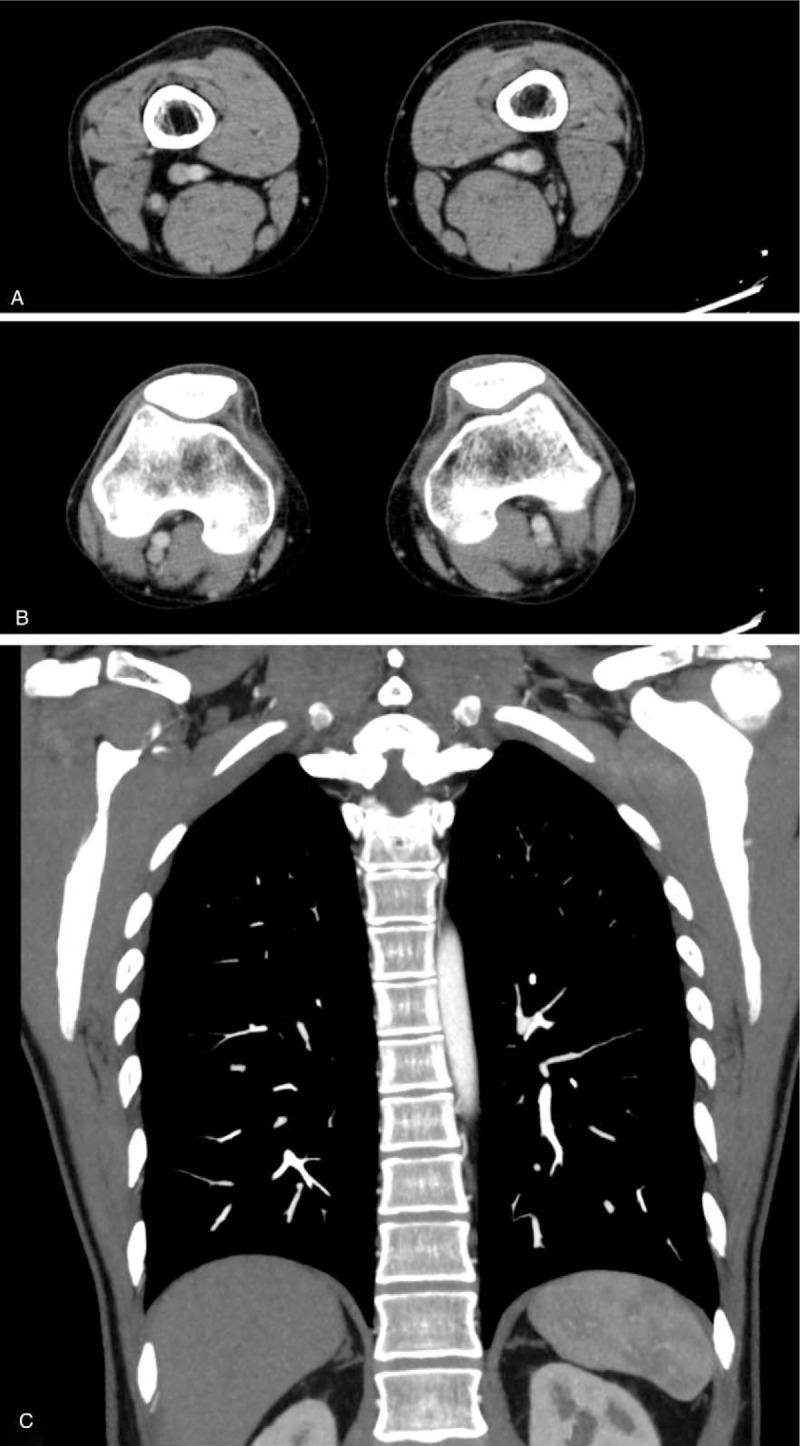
Follow up computed tomography which was performed approximately 1 month later revealed disappearance of thrombosis in the left femoral vein (A), left popliteal vein (B) and bilateral pulmonary artery (C).
